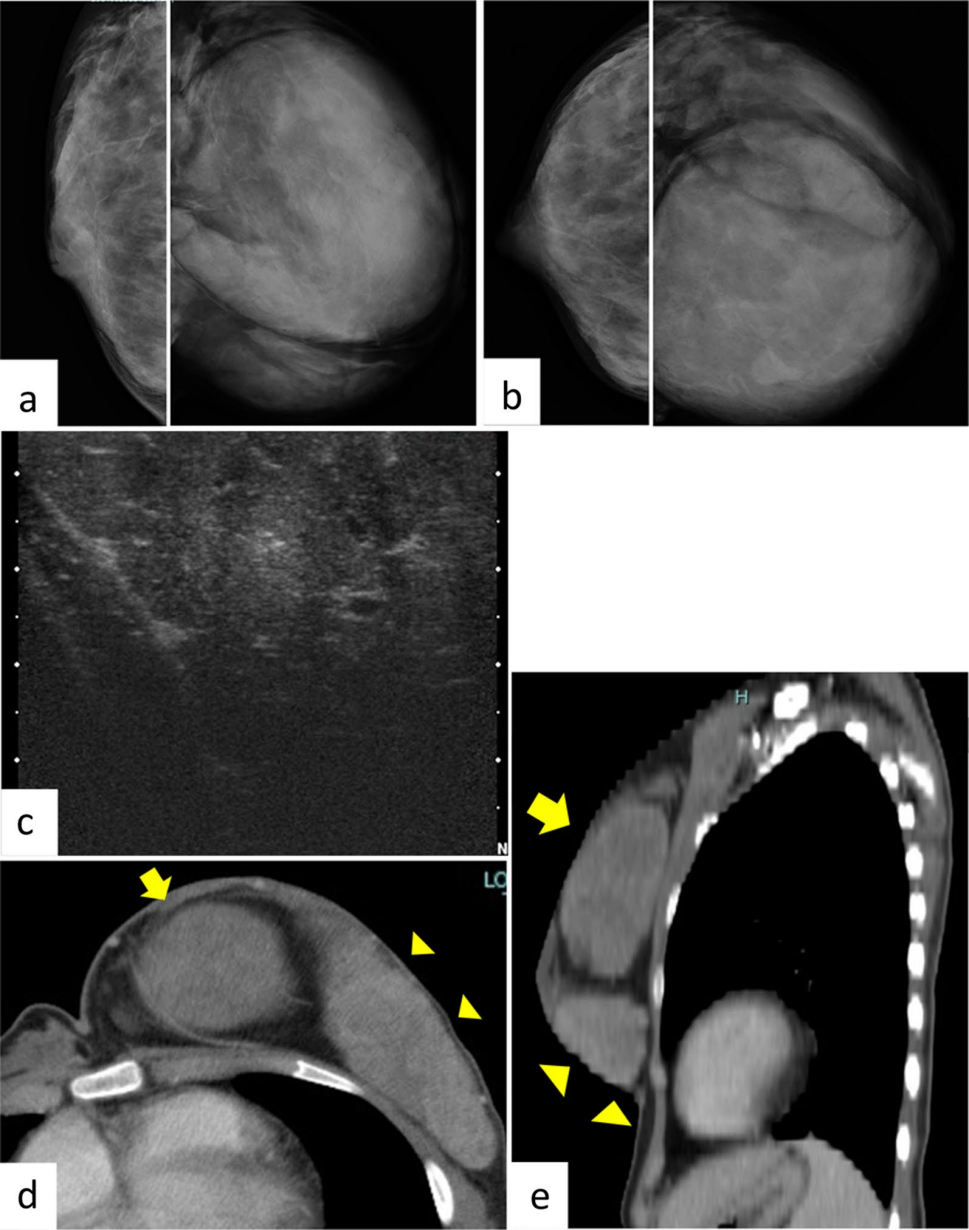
Imaging findings. a, b Mammography revealed a well-demarcated huge mass occupying the entire left breast (Breast Imaging Reporting and Data System category 3). a Medio-lateral oblique view; b cranio-caudal view. c Ultrasound showed two giant, well-demarcated, isoechoic lesions in the left breast, with homogeneous tumor parenchyma. d, e Contrast-enhanced computed tomography showed two well-demarcated masses in the left breast. Arrow indicates upper-inner lesion; arrowheads indicate lateral lesions. d Axial view; e sagittal view
